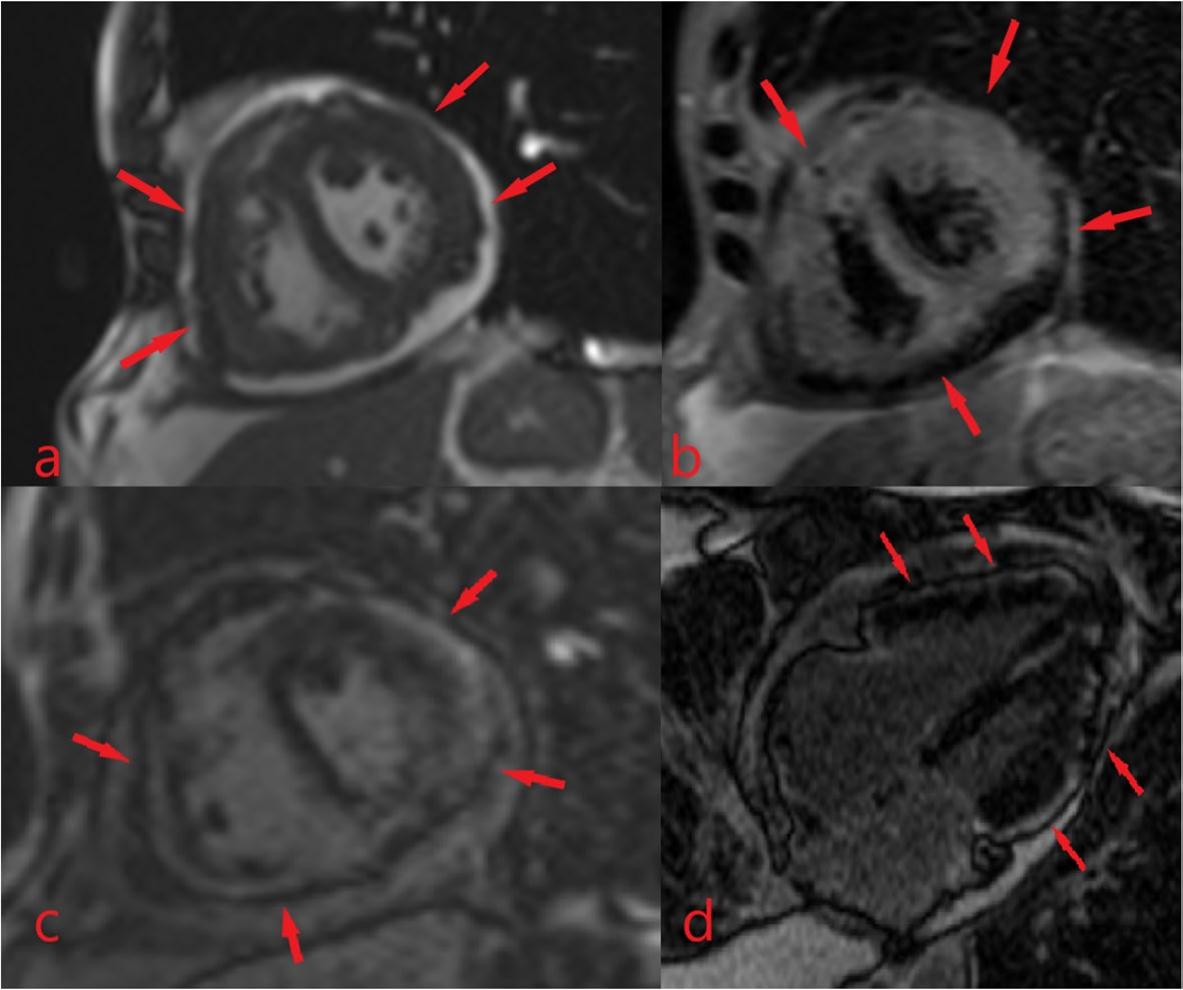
Cardiac magnetic resonance imaging shows 4a: Diffuse subepicardial infiltrative lesion in Cine image with 4b: increased T2 weighted signal intensity in Short tau inversion recovery image.4c and 4d: in late gadolinium enhancement images there is a diffuse subepicardial enhancement in both LV and RV myocardium
